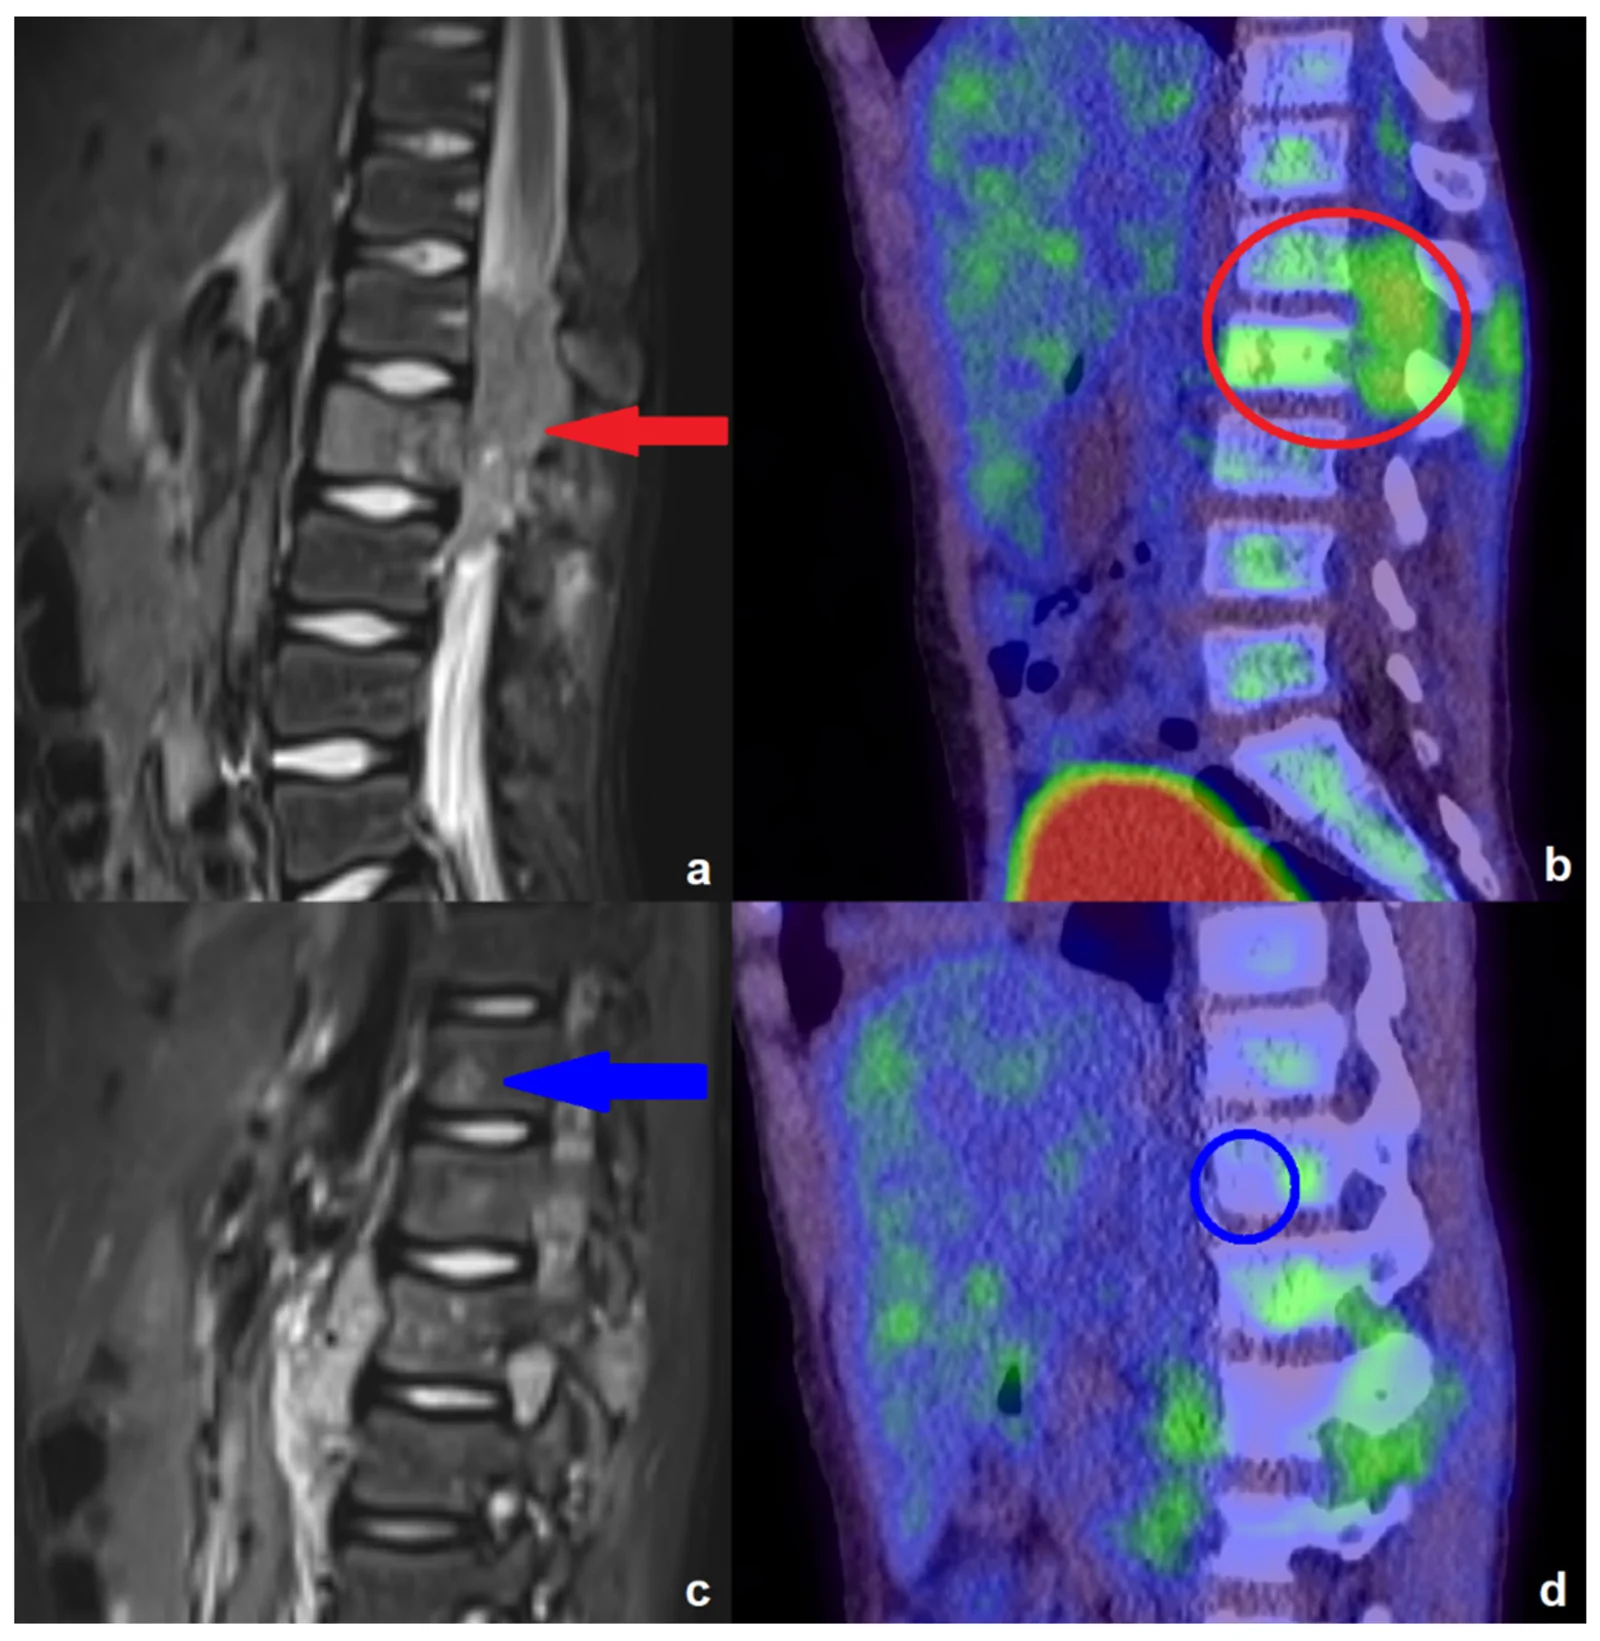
MR image (sagittal TIRM sequence) demonstrating a Ewing sarcoma originating from the lumbar spine with intraosseous and intraspinal growth (red arrow, (a)) with a low FDG uptake on the corresponding PET/CT image (color-coded as in Figure 2, red circle, (b)). A metastatic lesion in the lower thoracic spine (confirmed by complete regression under chemotherapy during follow-up) was only visible on the MRI (blue arrow, (c)) but not on the PET/CT scan (blue circle, (d)) due to missing FDG uptake.
